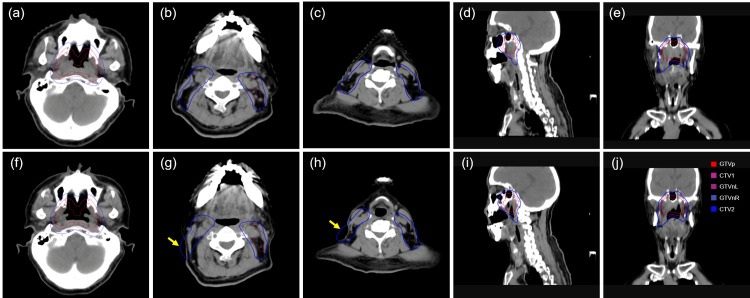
CT images show contour of target volumes in axial, sagittal, and coronal views (a-e) CT images acquired before radiotherapy show pre-radiotherapy target volume contours. (f-j) CT images acquired during the online ART fraction show contours rigidly registered from the pre-radiotherapy CT images. Target contours with GTVp in red, GTVn_L in purple, GTVn_R in light blue, CTV1 in scarlet, CTV2 in blue. CT: Computed tomography; ART: Adaptive radiotherapy; GTVp: Gross tumor volume at the primary site; GTVn_L: Left involved cervical lymph node; GTVn_R: Right involved cervical lymph node; CTV1: High-risk clinical tumor volume; CTV2: Low-risk clinical tumor volume
